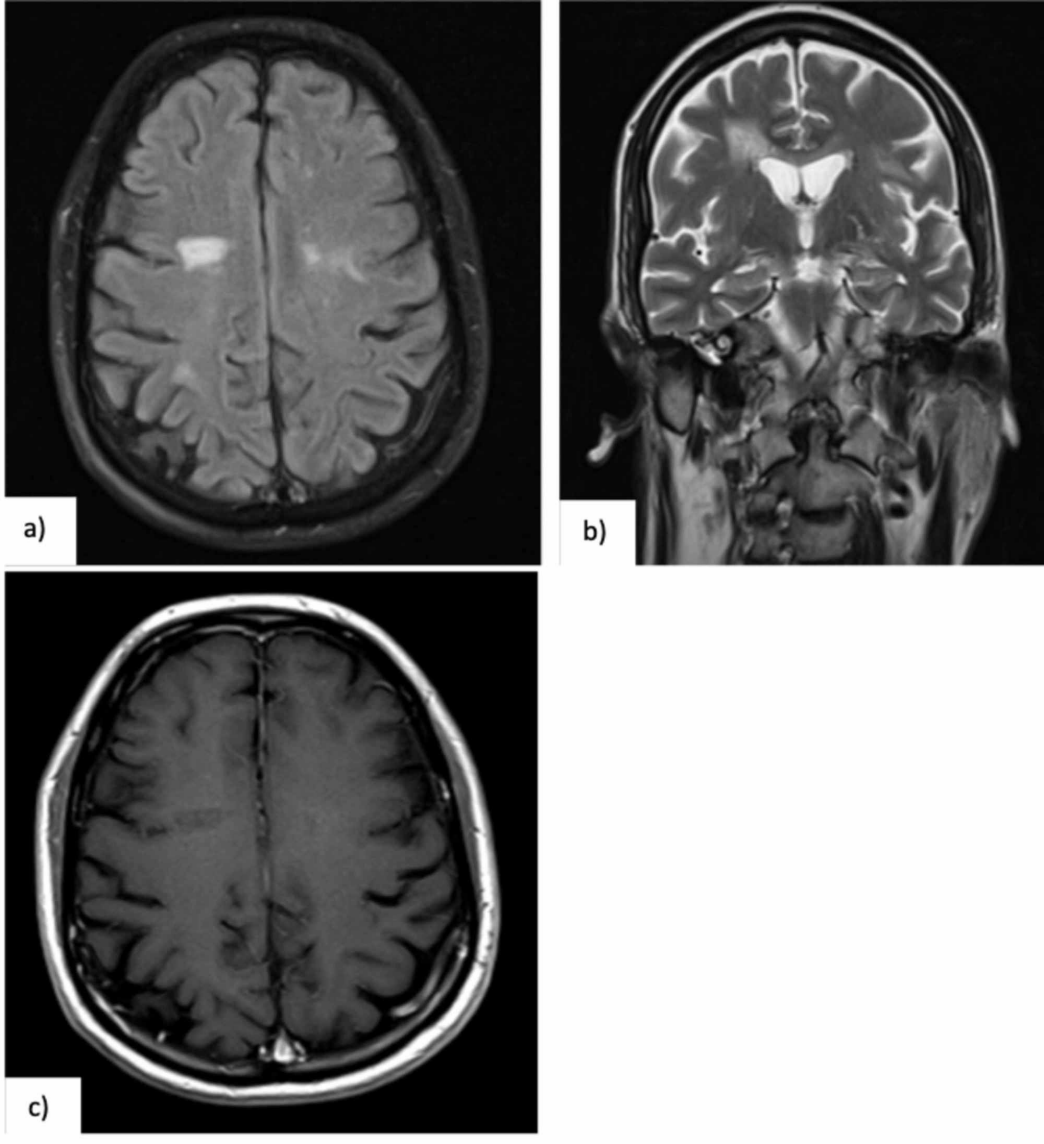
(a, b) Brain MRI (04-06-2018) performed one year after clinical presentation, showing remarkable reduction of previous lesions in T2 FLAIR (axial) and T2 TSE (coronal) and sequences, and (c) almost no perceptible T1 hypointensities (axial, after gadolinium injection).
